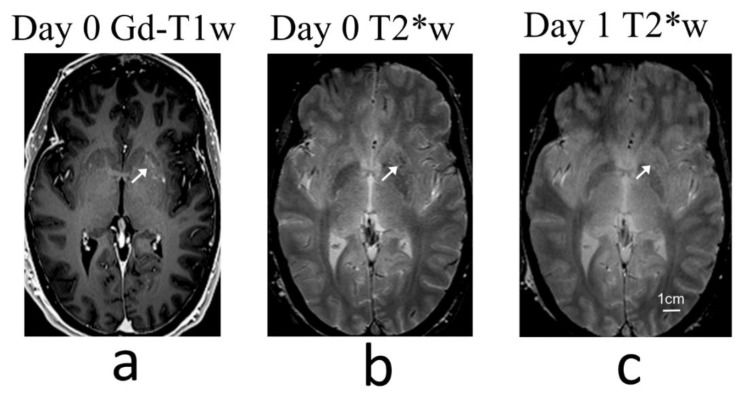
MRI summary of treatment session 1 in patient 1 (cavitation dose target = 0.40). (a) Gd-enhanced T1-weighted MR image shows BBB opening within the left putamen (arrows). (b) T2*-weighted MR images acquired immediately post-treatment revealed a small number of hypointense pixels within the target volume that were constrained within the axial slice shown. (c) Corresponding T2*-weighted MR image acquired one day post-treatment no longer contained regions of signal hypointensity within the targeted putamen.
